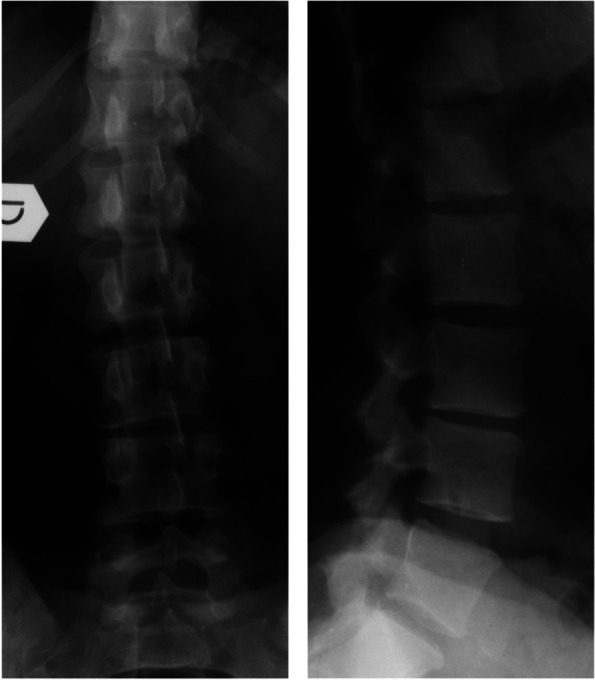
X-rays of the patient at the first consult in June 2002
